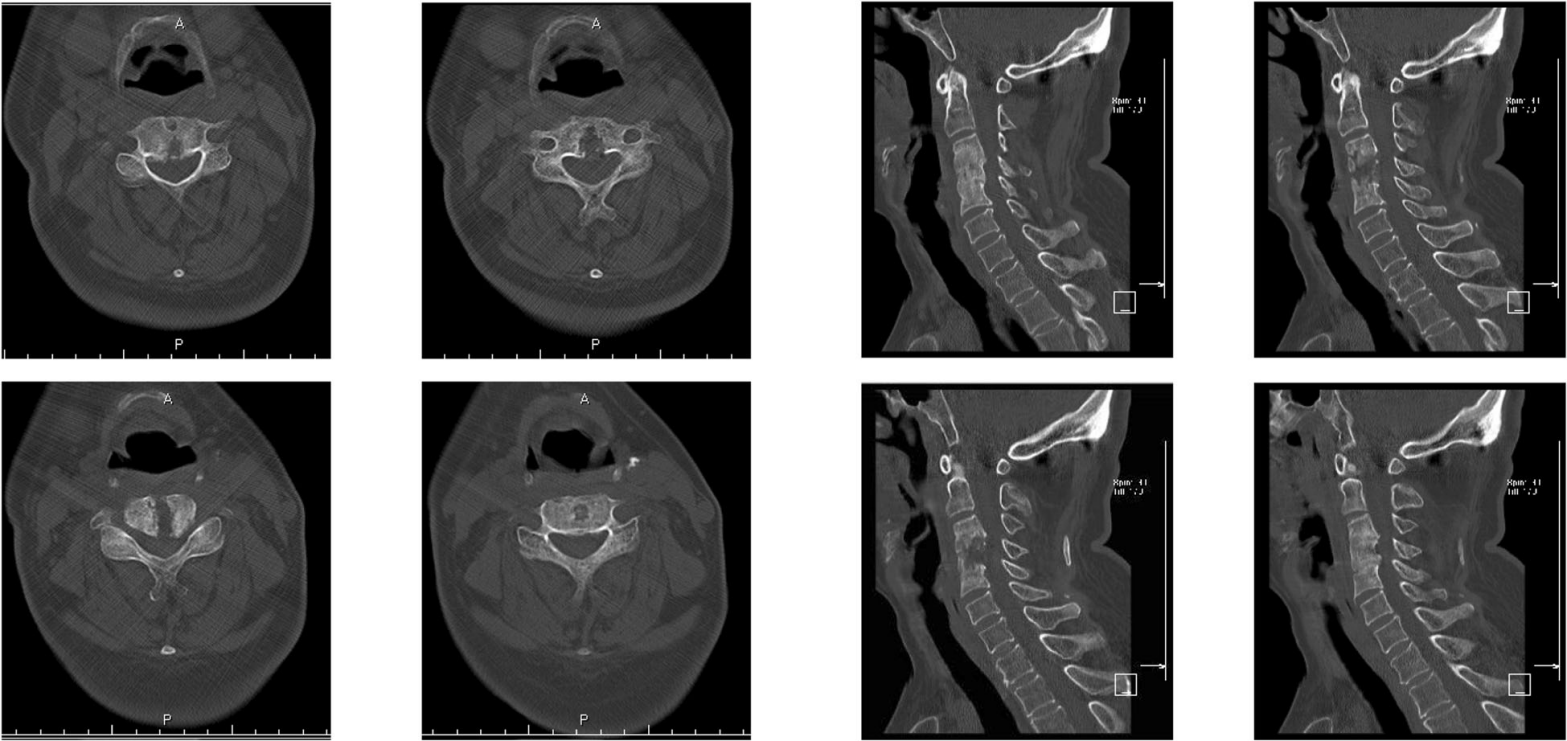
CT and MRI images 3 months after application of Hallo frame support
